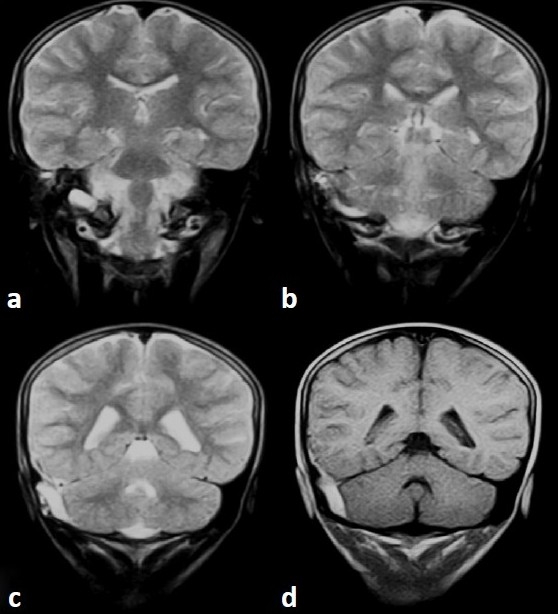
MRI T2W image (a–d) showing hyperintense area in the region of right sigmoid sinus
